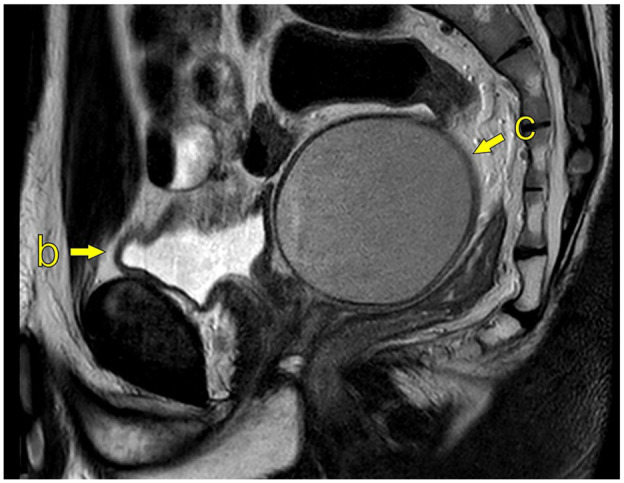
MRI of the pelvis; 1.5T Philips Ingenia (Philips Medical System), T2W sagittal image (TR 4000/TE 80). (b) Bladder, (c) Cystic enlargement of left seminal vesicle, measuring 7.2 × 6.7 × 9.1 cm (ca. 219 mL).
